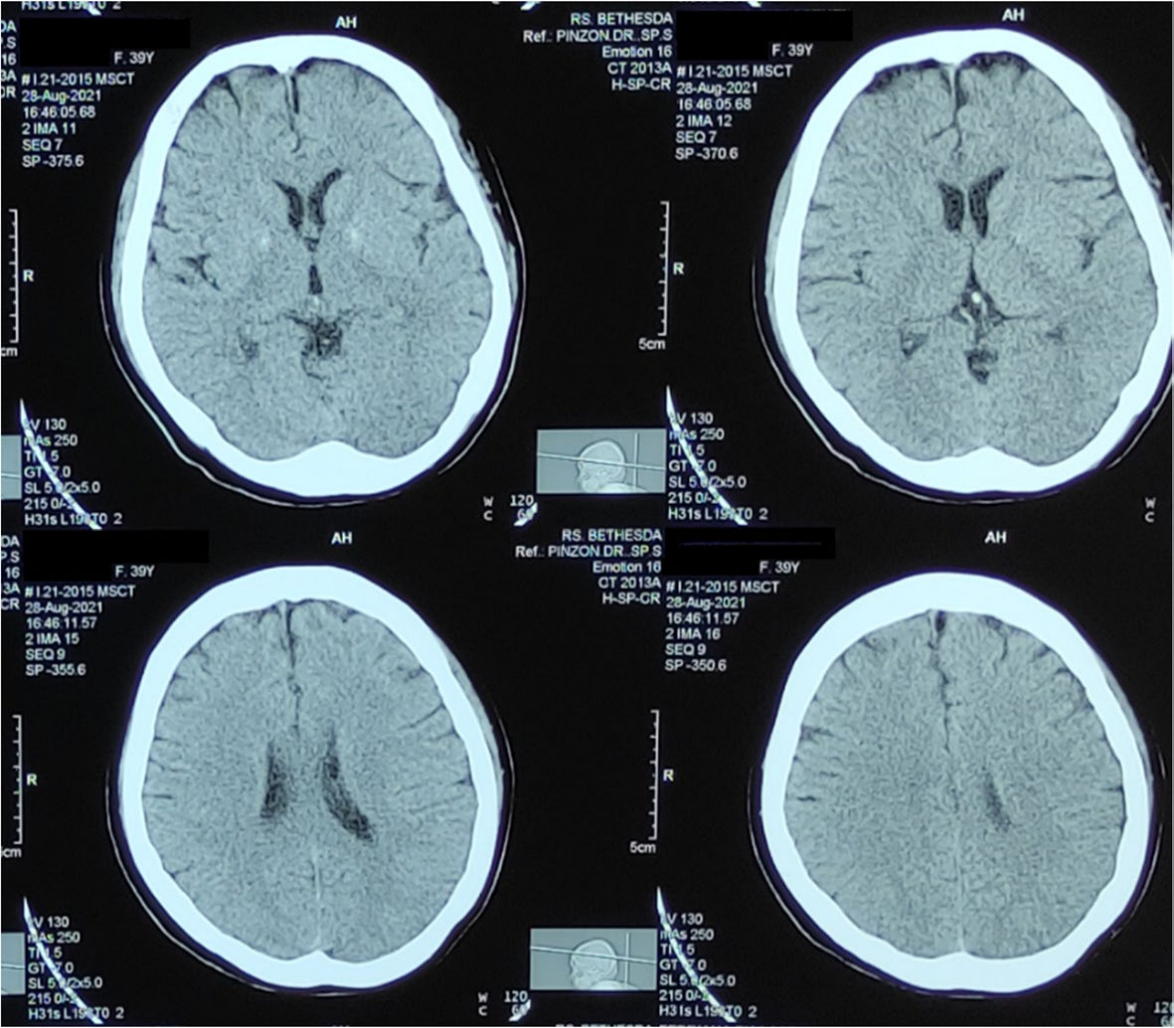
Head CT-scan examination shows no abnormalities
